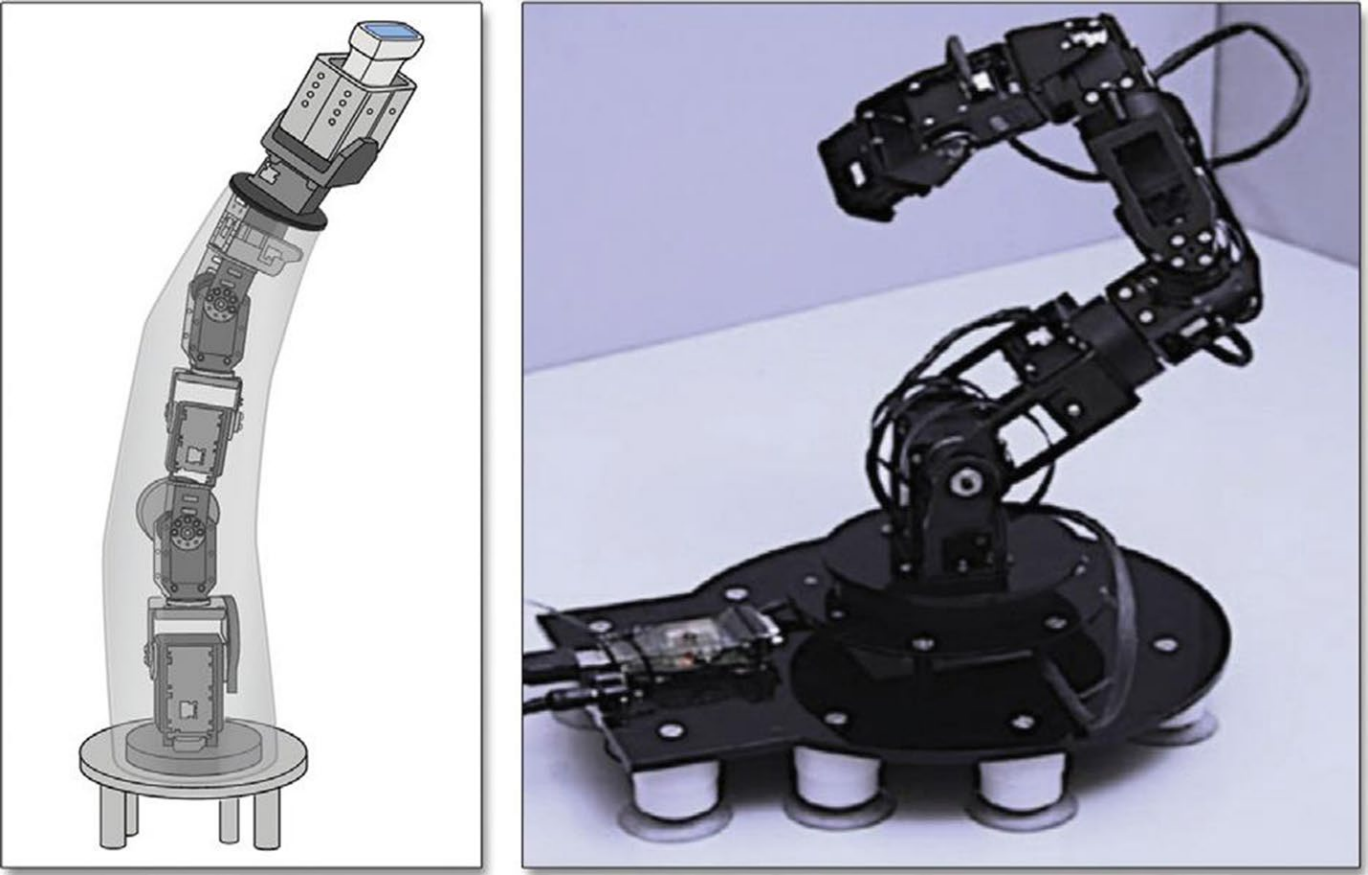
Robotic arm for slave station of telerobotic ultrasonography platform. Reprinted with permission from [122]
